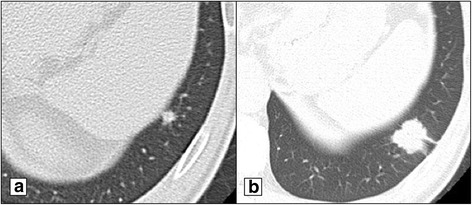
Imaging studies of the first pulmonary metastasis. Chest CT (53 months after curative resection of the primary pancreatic tumor) shows a tiny nodule at the lower lobe of the left lung (a). Follow-up CT (70 months after curative resection of the primary pancreatic tumor) shows that this nodule has enlarged to 2 cm in size with spiculation (b). It is difficult to determine whether this nodule is a primary or metastatic tumor on imaging
